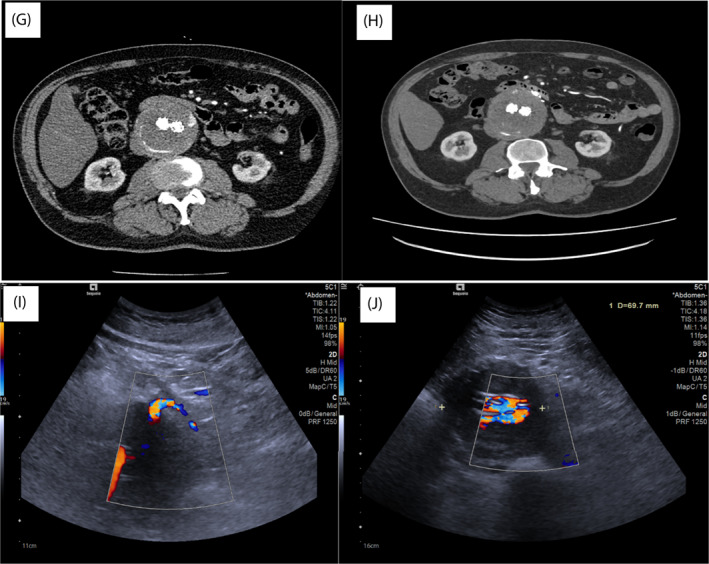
(G) Preoperative CTA with persistent T2E. (H) Postoperative CTA with type II endoleak (T2E) resolved. (I) Preoperative CEUS with inferior mesenteric artery (IMA) filling of sac. (J) Postoperative CEUS with resolved IMA filling
